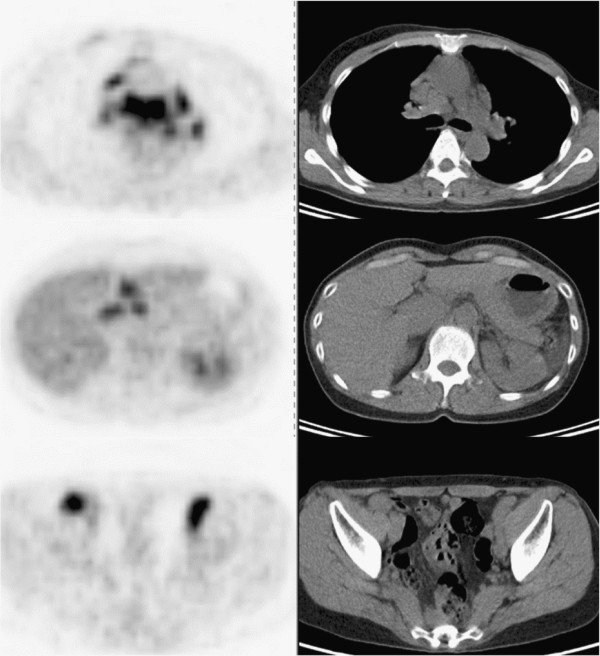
Case 4. Axial FDG-PET/CT images obtained 4 years after antineoplastic therapy for left breast cancer (left column, FDG-PET; right column, CT).
